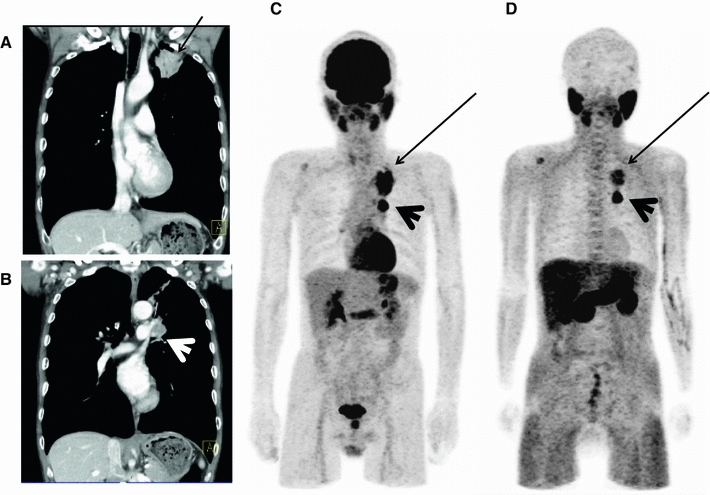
A case of lung cancer. Fifty-nine-year-old asymptomatic male patient. Screening chest X-ray showed abnormal density in the left upper lung zone. The patient underwent a contrast-enhanced chest CT scan, which revealed a nodule in the left apex (a) with probably lymph node (ln) metastasis in the *left* hilum (b). Bronchoscopic biopsy revealed squamous cell carcinoma (SCC). Both ^18^F-FDG PET and ^11^C-MeAIB PET (c, d: MIP image) showed high accumulation in the left upper lobe lesion (SUVmax = 13.2 for ^18^FDG, 6.3 for ^11^C-MeAIB, *arrows*) and *left* hilar ln (SUVmax = 21.9 for ^18^F-FDG, 11.5 for ^11^C-MeAIB, *arrowheads*). It should be noted that ^18^F-FDG uptake was relatively greater in the periphery than that of ^11^C-MeAIB. It was confirmed as concomitant inflammatory changes in the tissue surrounding the lesion. A small bone metastasis was also suspected in the right scapula (not pathologically confirmed)
